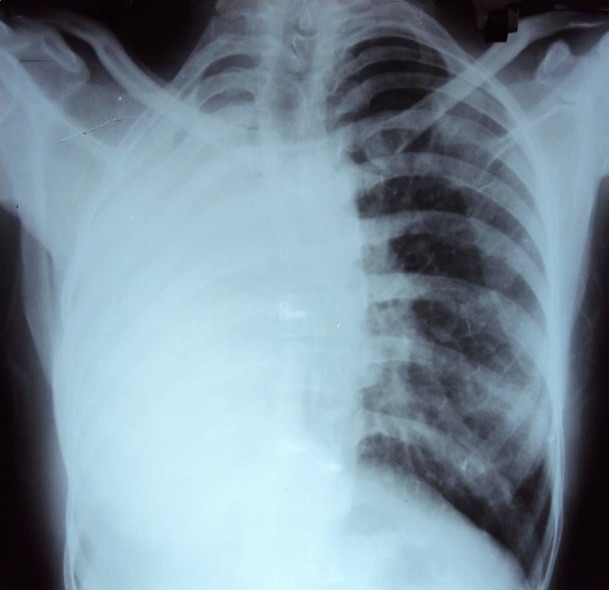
Chest radiography, showing opaque right hemithorax with the crowding of the ribs and mediastinal shift to right.
